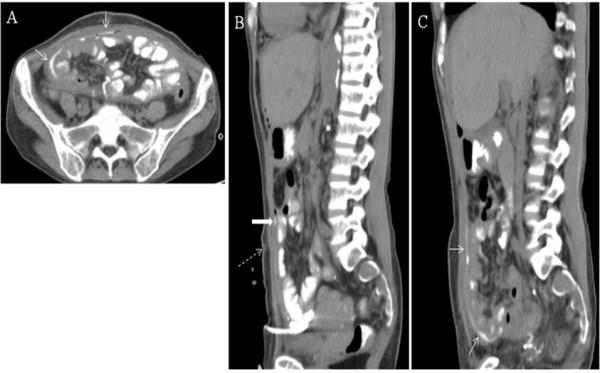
A, B and C. Peated CT scan when pus was completely drained and patient ingested 400 ml of Ultravist® medium. The transverse image suggested a cocoon-like conformation which kept the leaking medium from entering the peritoneal cavity (arrow in Figure
